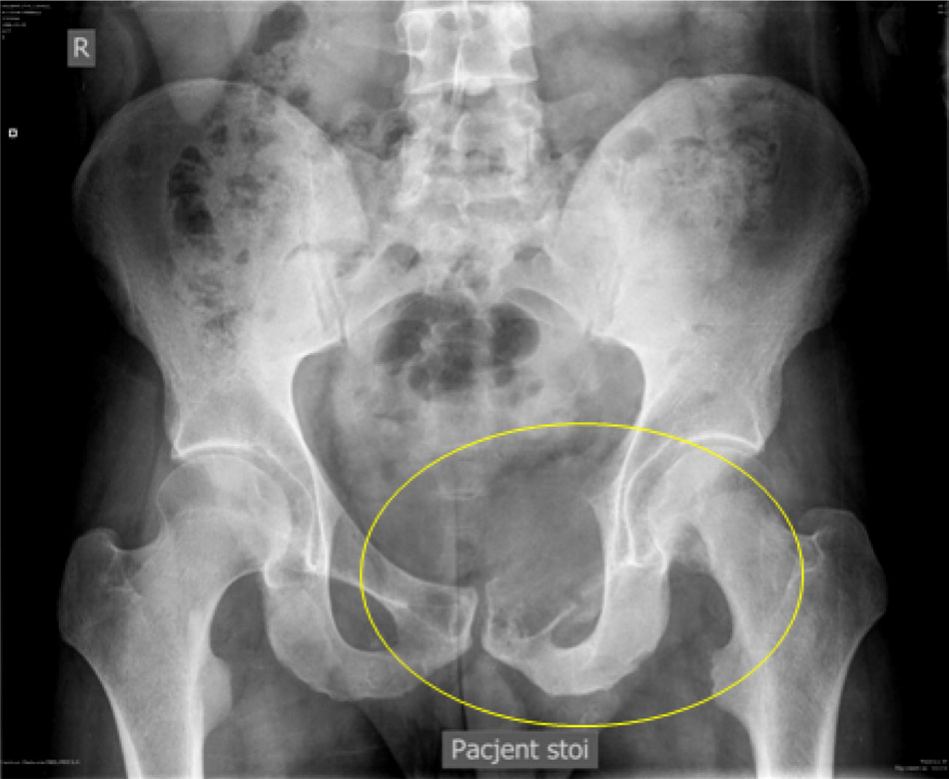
Case 4: x-ray of pelvis: large osteolytic lesion, deforming entire right pubic bone
